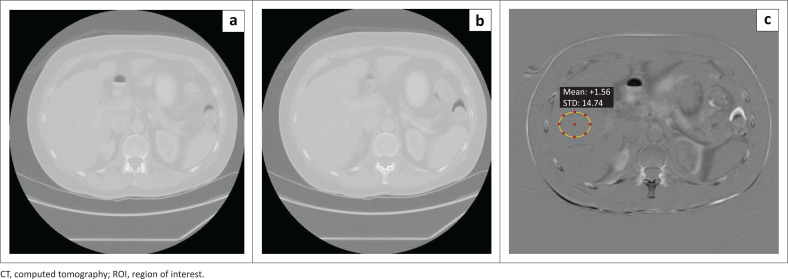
An example of the subtraction process for image quality analysis. (a) and (b) are adjacent images, and therefore independent images in the CT dataset. The difference between (a) and (b) is given as (c). On the subtracted image (c), an elliptical ROI is placed on the liver providing the mean and standard deviation in Hounsfield units.
